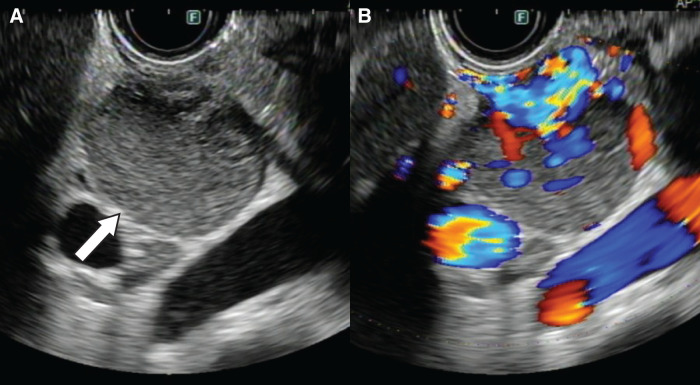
EUS findings. (A) A well-defined, homogeneous internal round tumor can be seen adjacent to the duodenal bulb (white arrow). (B) The tumor showed good blood flow on color Doppler. EUS, endoscopic ultrasonography
